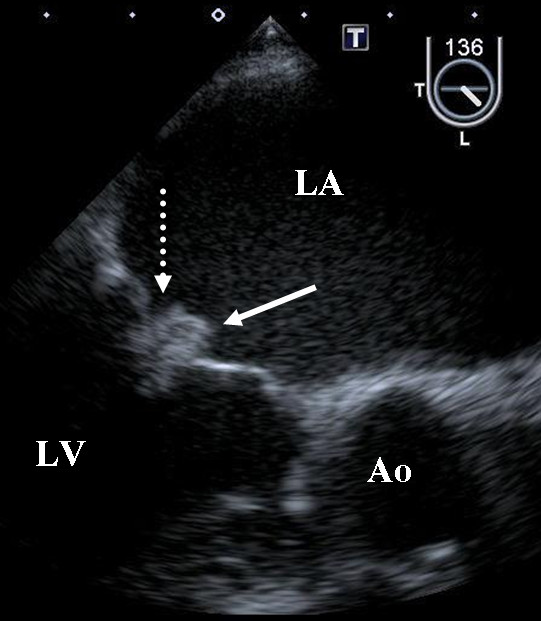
Masses of the mitral valve. Ao, aorta; LA, left atrium; LV, left ventricle. Transesophageal echocardiography showed mobile, echo-dense masses measuring 3 × 7 mm and 6 × 4 mm attached, respectively, to the anterior (white arrow) and posterior (dotted white arrow) mitral valve leaflet.
